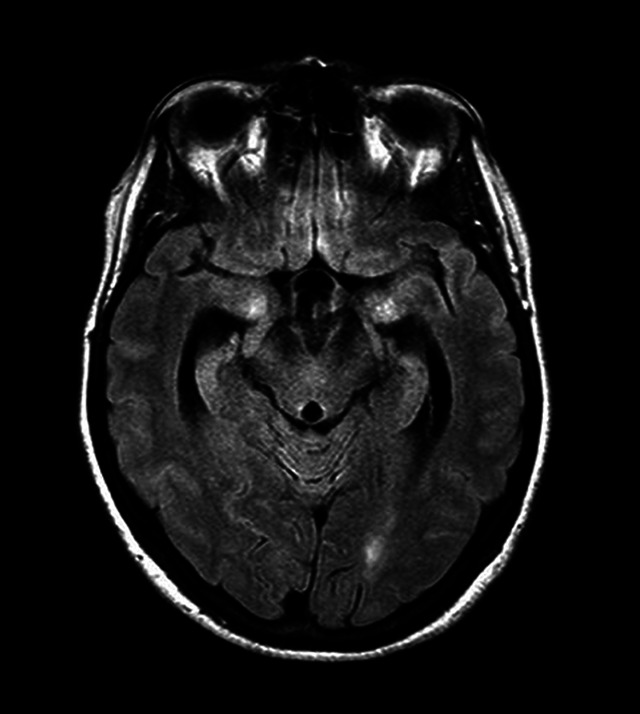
Axial view on the FLAIR sequence (*Fluid-Attenuated Inversion Recovery*) showing cerebral aqueduct dilation, characterized as *flow-void*.
